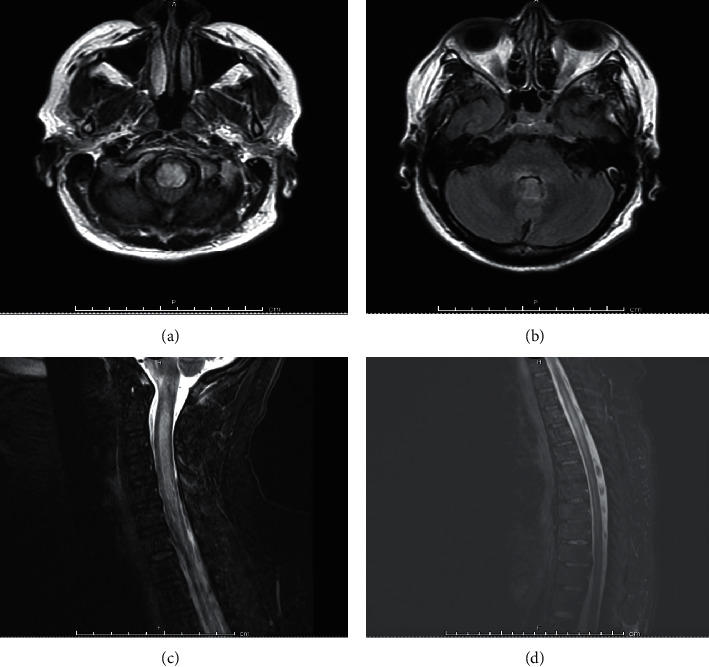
FLAIR brain MRI of a 48-year-old Hispanic woman with SLE with noted medullary lesion extending to the periaqueductal gray matter (a, b) and with diffuse lesions in the C and T spine (c, d).
